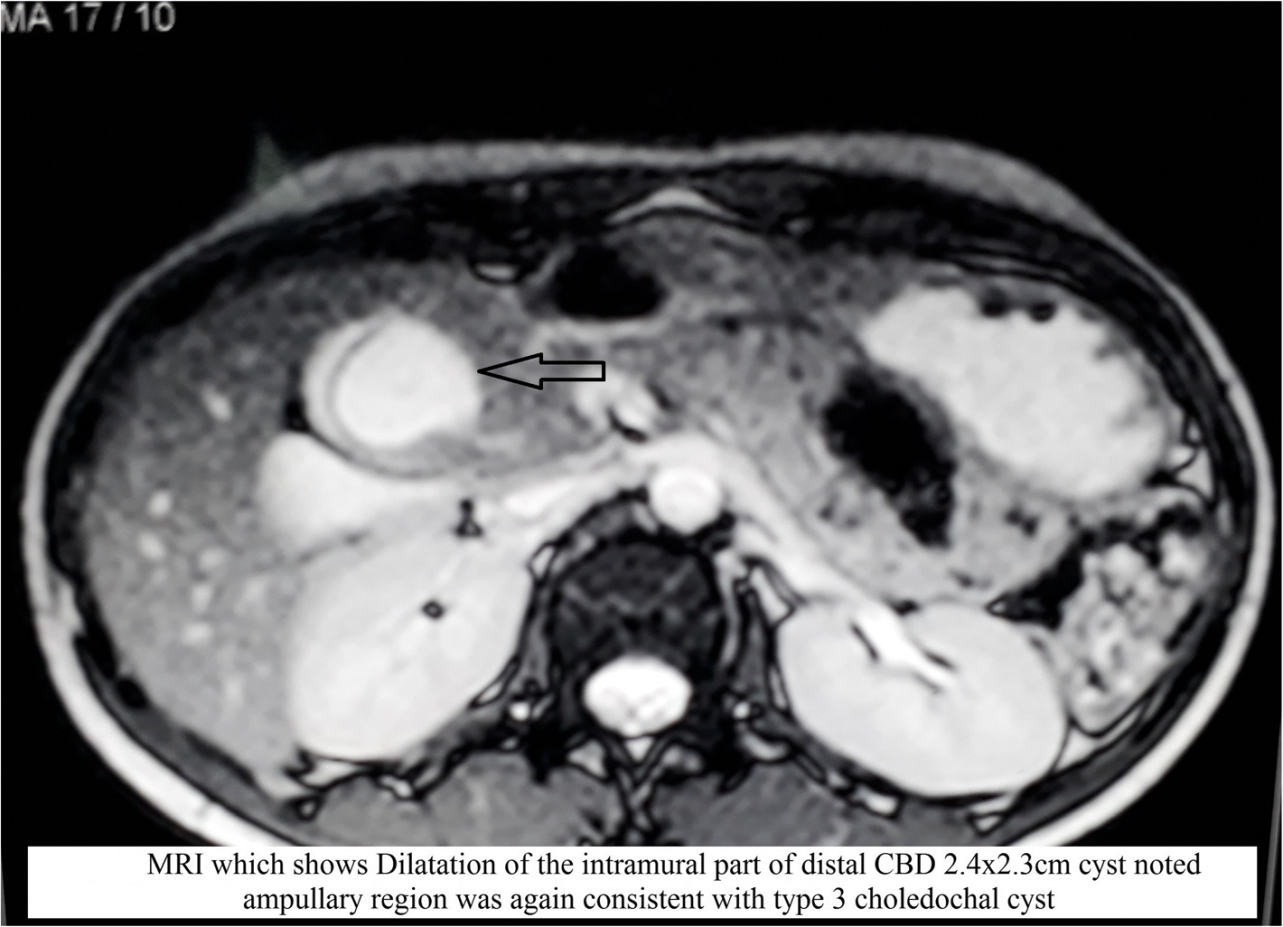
MRI abdomen showing dilatation of the intramural part of distal CBD 2.4 x 2.3cm cyst (*arrow*) noted ampullary region suggestive of Type 3 choledochal cyst
